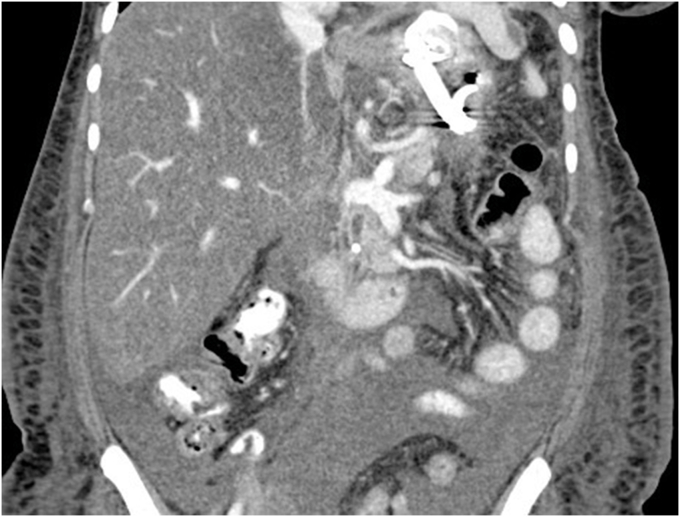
CT of abdomen and pelvis showing resolution of walled-off necrosis cavity.
